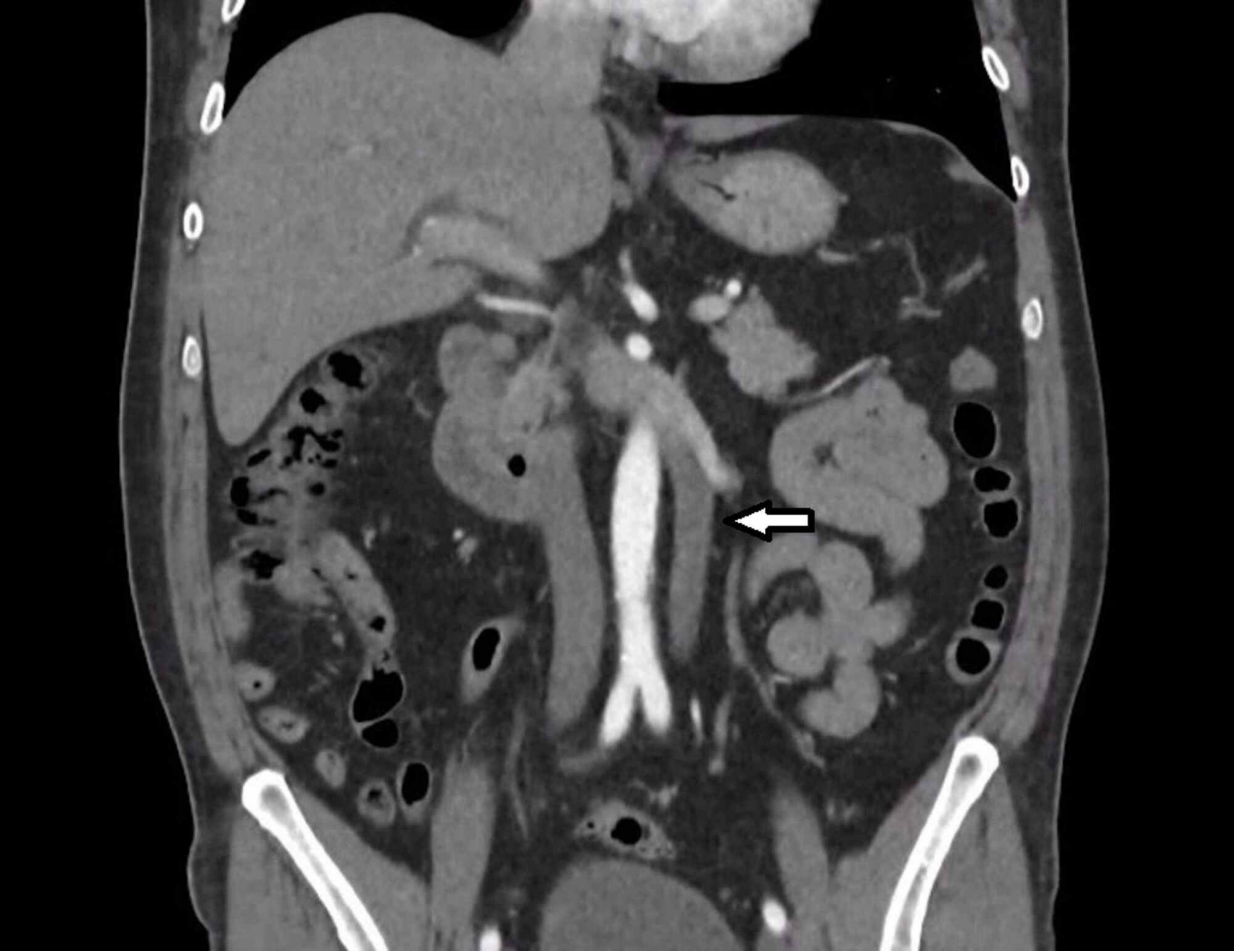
CT abdomen (coronal): Bilateral vena cava merging into the preaortic trunk.
